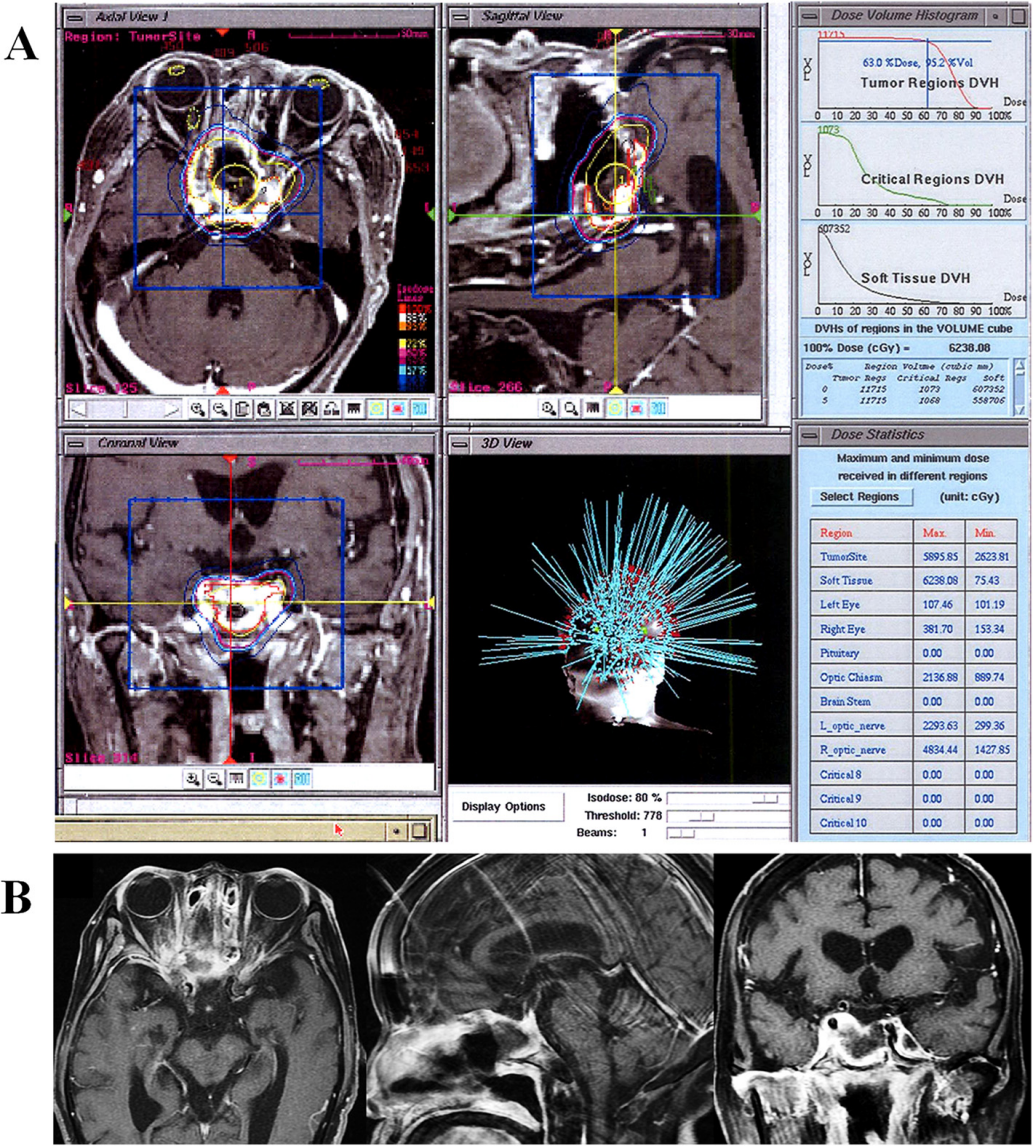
A. Planning of CyberKnife^® ^radiation. The planned radiation was applied in five fractions, mainly to the residual tumor in the sphenoid and ethmoid sinuses including the optic canals. DVH: dose volume histogram. B. Follow-up magnetic resonance imaging performed 5 months after CyberKnife^® ^treatment. The residual tumor exactly shrinks after CyberKnife^® ^radiation. Of note, the size of enhanced lesions in the cavernous sinuses has decreased remarkably.
